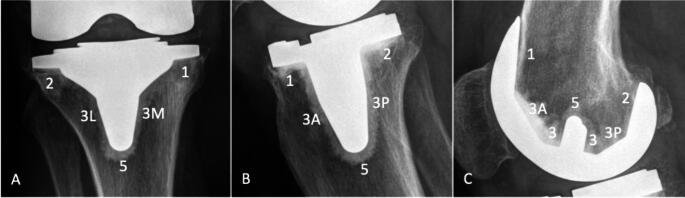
Radiographic assessment of radiolucent lines based on the “Modern Knee Society Radiographic Evaluation System and Methodology for Total Knee Arthroplasty [23]”: (A) anteroposterior radiograph of the tibial component (3M: medial side of the keel; 3L: lateral side of the keel); (B) lateral radiograph of the tibial component (3A: anterior side of the keel; 3P: posterior side of the keel); (C) lateral radiograph of the femoral component (3A: anterior chamfer cut, 3P: posterior chamfer cut)
